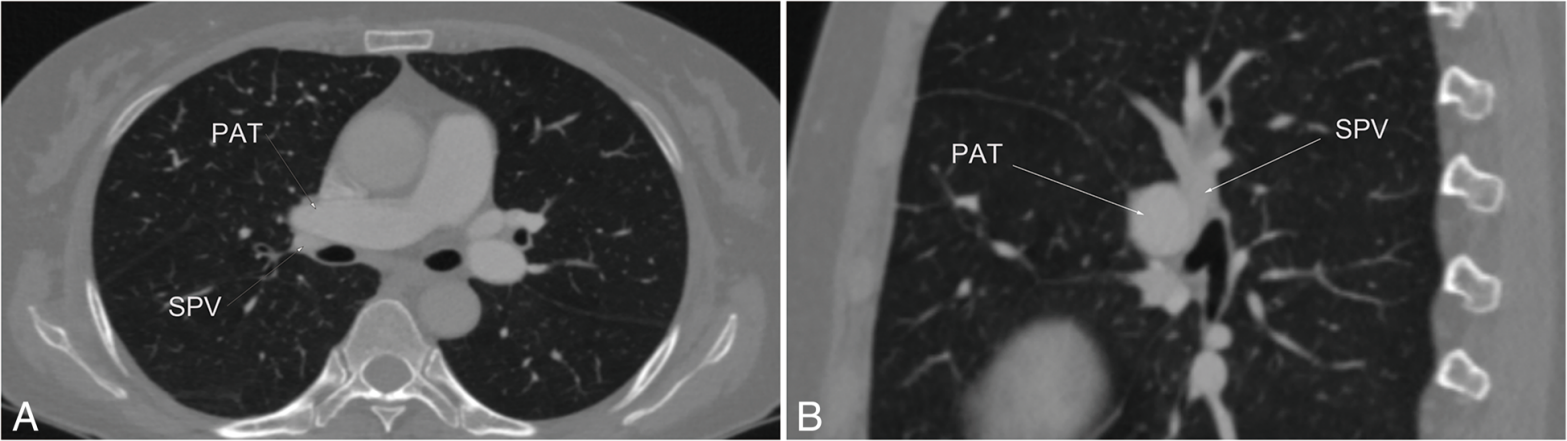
Contrast-enhanced three-dimensional computed tomography showed that the superior pulmonary vein abnormally ran between the pulmonary artery trunk and the right main bronchus
